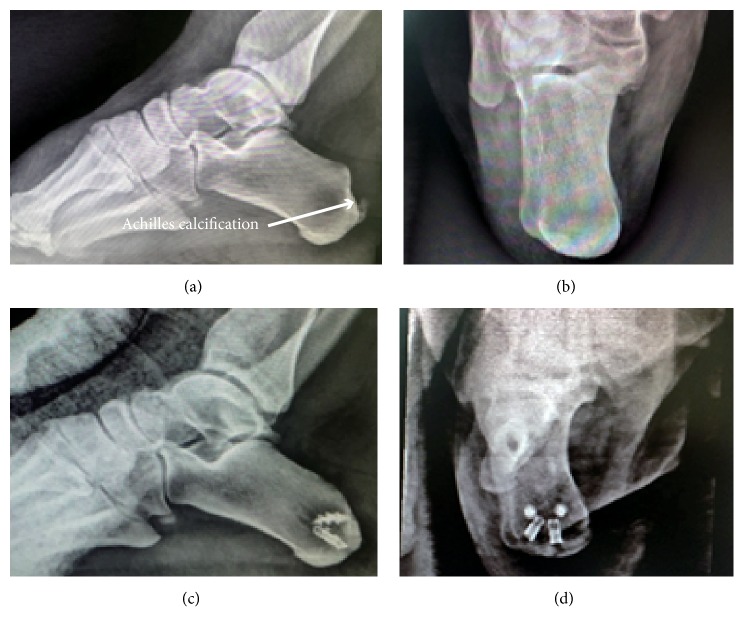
Case 3, a 40-year-old woman showed right posterior heel pain for 3 years, and her nonsurgical treatment was ineffective*. (a) Preoperative radiograph revealed calcification near the posterior calcaneus. (b) Axis X-Ray plain film of calcaneus before operation. (c) Postoperative radiograph showed calcification in the Achilles tendon insertion had been removed, and the Achilles tendon was fixed by double row suture bridge. (d) Postoperative calcaneal axis X-Ray film showed the distribution of inner and outer anchors.
